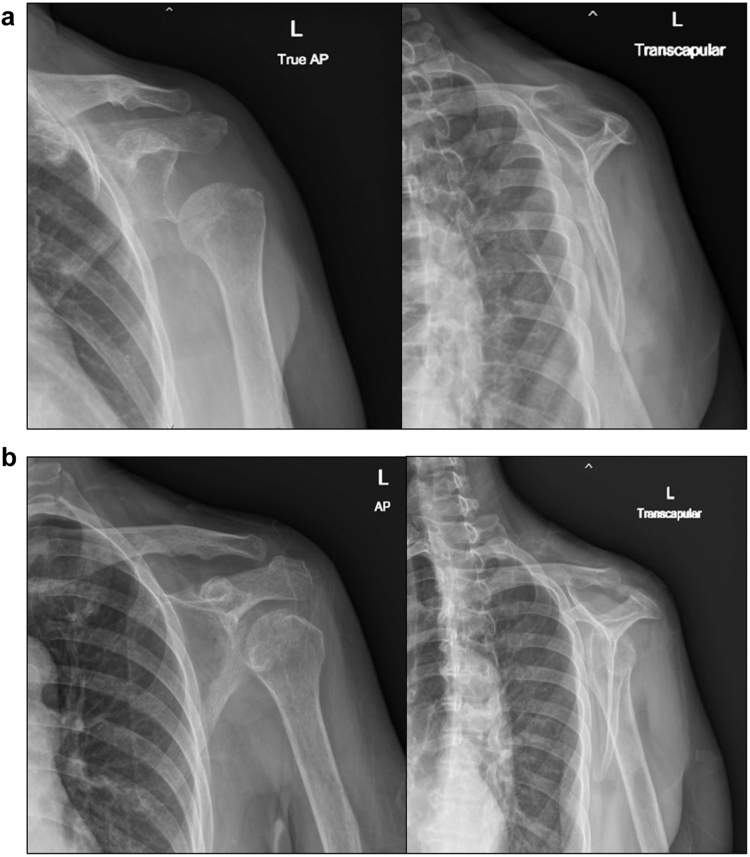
(a) Pre-reduction plain radiograph showing inferior subluxation of the *left* humeral head. (b) Postreduction plain radiograph confirming restored glenohumeral alignment. *AP*, anteroposterior.
